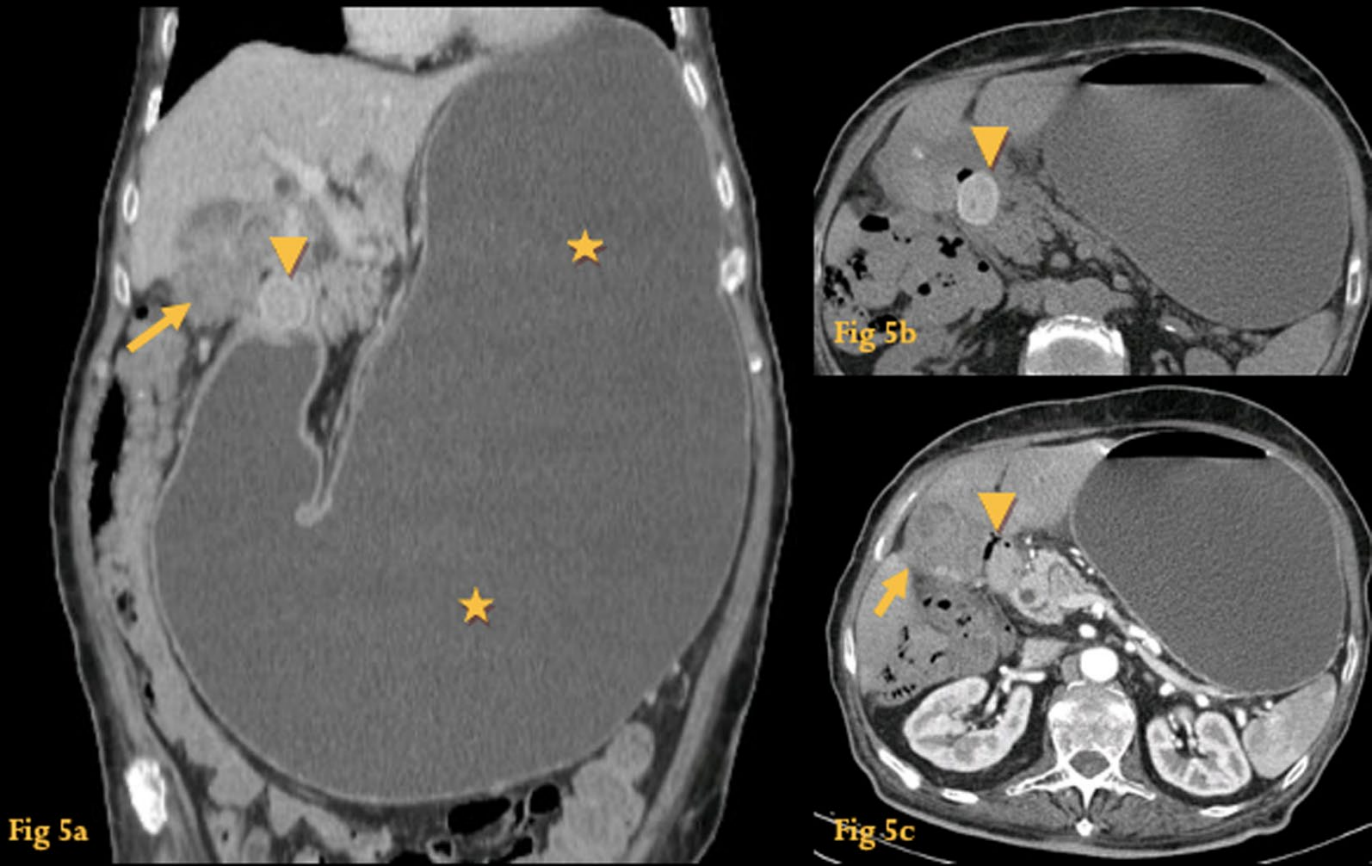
Bouveret syndrome. CT coronal reformation (a) shows marked gastric dilatation (asterisks) caused by a round lesion at the duodenal bulb (arrowhead); there is also densification and indefinition of the contour of the gallbladder (arrow). Unenhanced CT (b) shows the lesion is spontaneously hyperdense-endoluminal gallstone at the duodenal bulb (arrowhead). A small fistulous tract filled with air (c) (arrowhead) is seen between the duodenal bulb and the expected location of the gallbladder, which had ruptured and formed a phlegmon (arrow)
